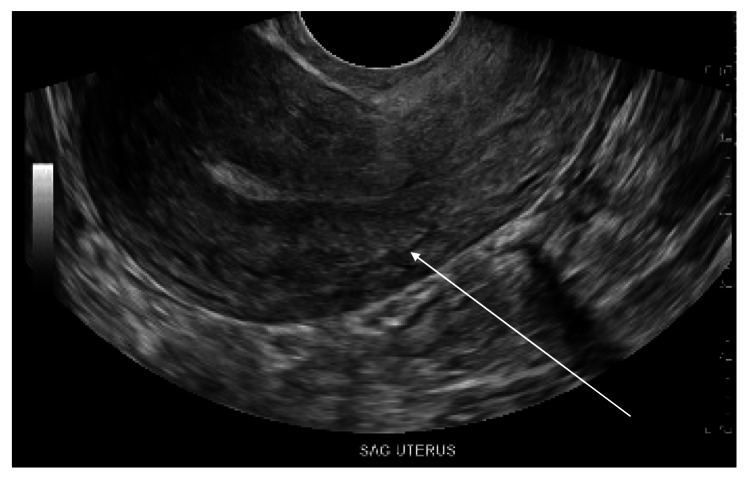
Transabdominal ultrasound demonstrating sagittal view of uterus with no intrauterine device seen (uterus denoted by the white arrow).
